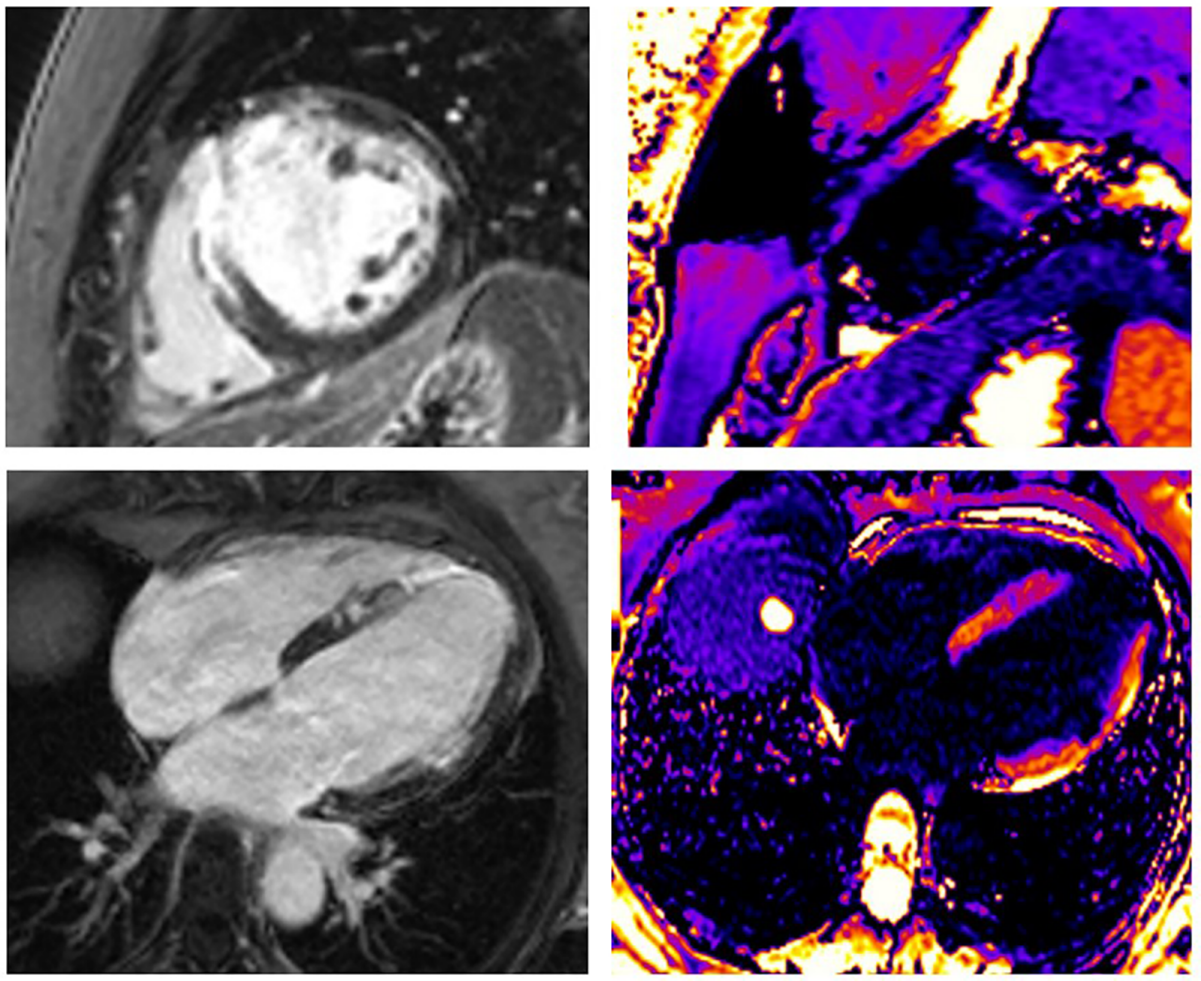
A 43-year-old woman presented as STEMI anterior localization, SCAD on left anterior artery type 3, and TIMI flow 0, treated with percutaneous coronary intervention (PCI) and implanted four stents guided with intravascular ultrasound (IVUS). LGE was seen in the medio-apical part of the septum, apical parts of the anterior wall transmurally, and in the medial parts of the anterior wall in the sub-endocardial layer. Using post-contrast T1 mapping, the zone of fibrosis, which was the zone of infarction, was also present in the same segments. The size of fibrosis (infarct size) was 17%.
